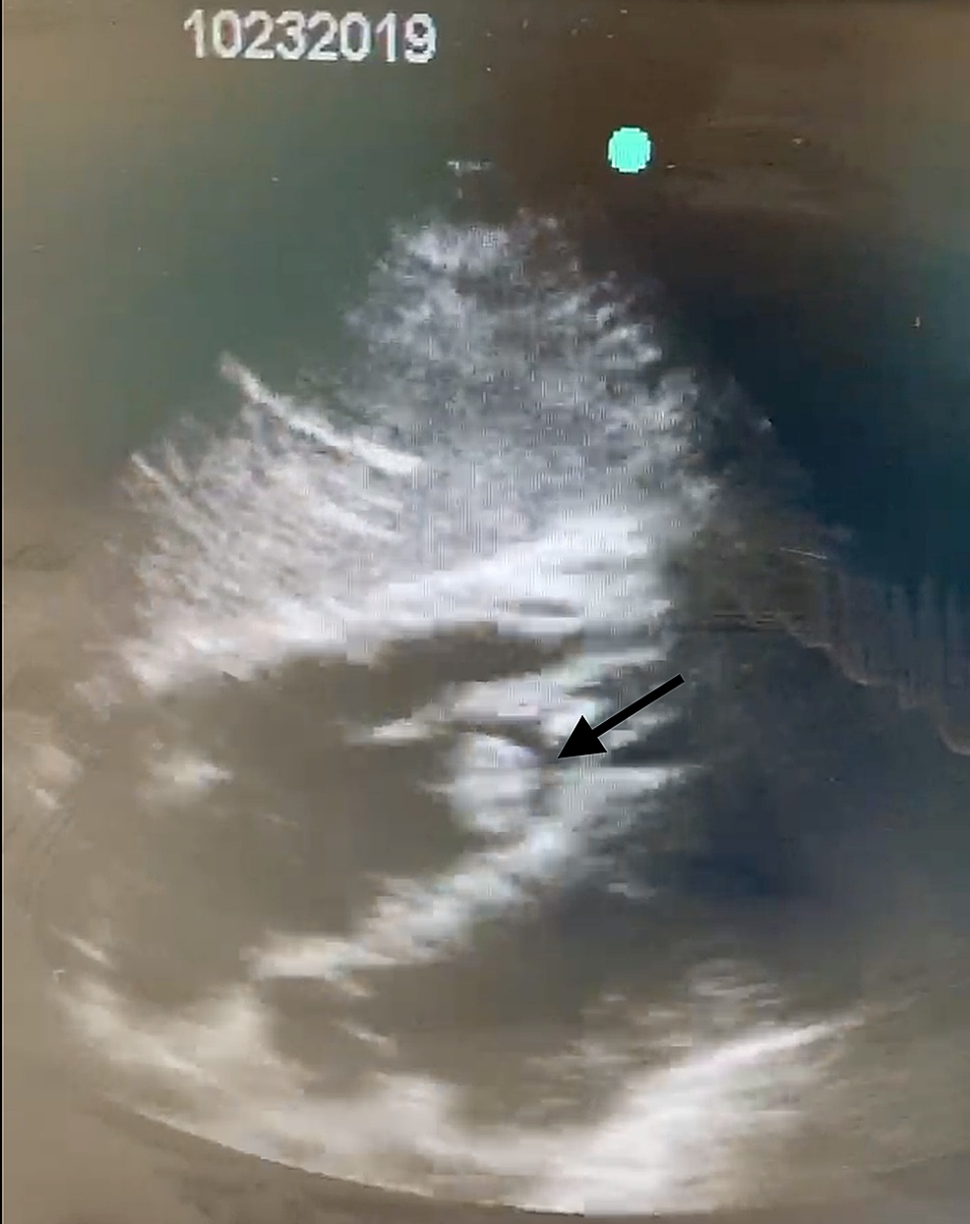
Point-of-care ultrasound of the heart showing thrombus in transit in the right ventricle (black arrow).
